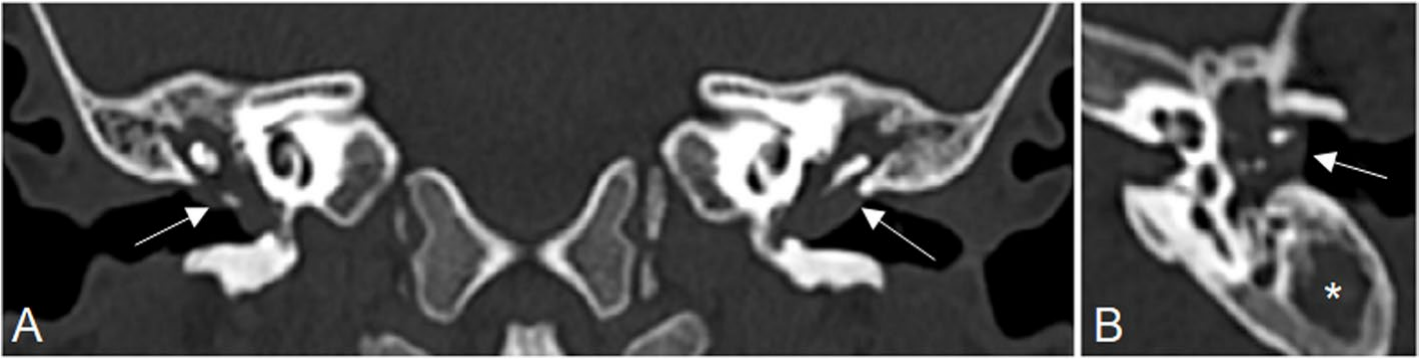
Temporal bone CT scan with (A) coronal and (B) axial reconstructions shows bilateral middle ear and mastoid effusion with complete opacification of the tympanic cavities (arrow) and mastoid air cells (asterisk)
